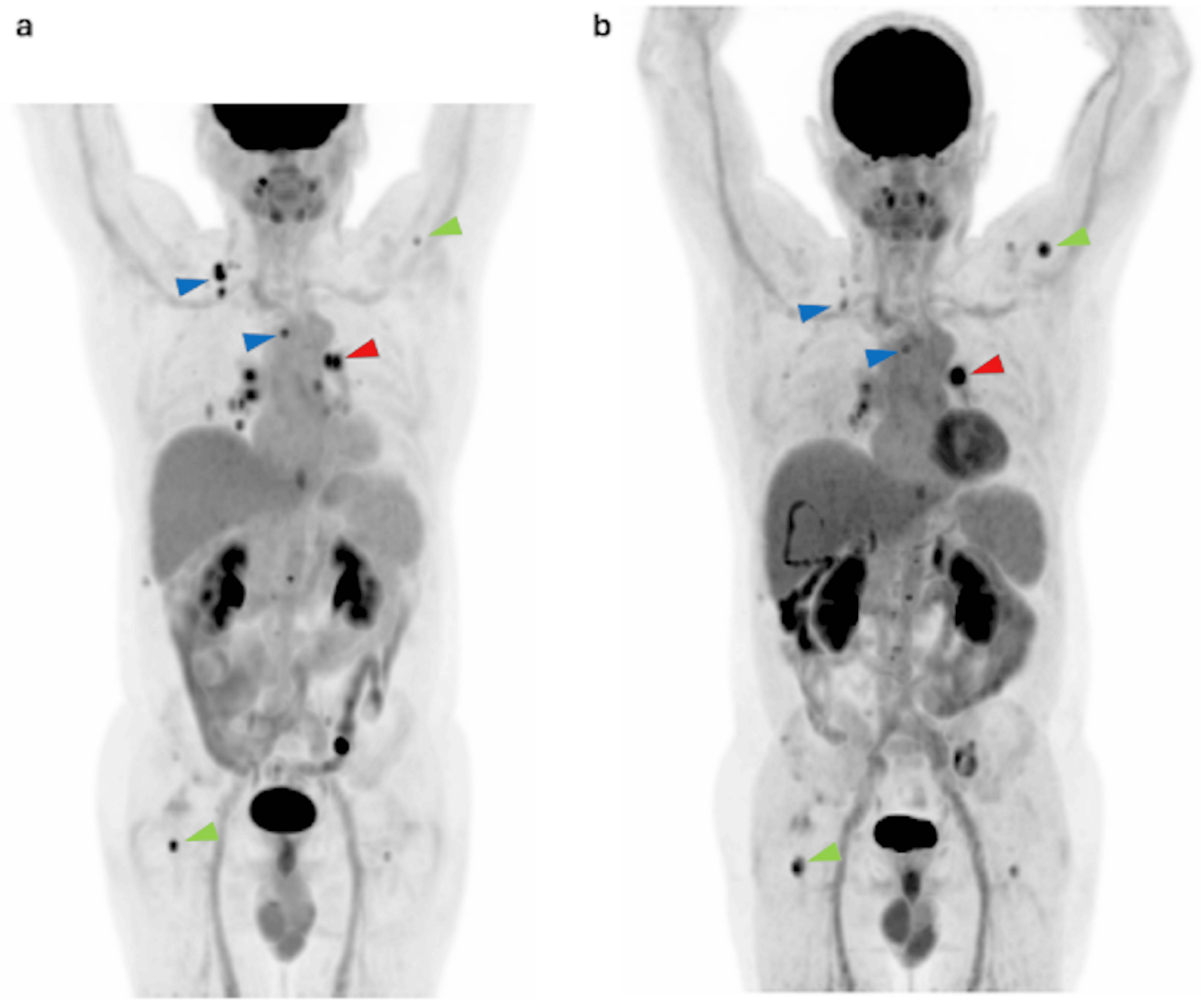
MIP images from FDG-PET/CT studies performed in June 2023 (a) and July 2024 (b). Interval metabolic progression is observed, characterized by increased FDG uptake in a left hilar pulmonary lymph node (red arrowhead) and multiple osseous lesions (green arrowhead), while several cervical and mediastinal lymph nodes show decreased tracer avidity, indicating a mixed metabolic response (blue arrowhead). MIP: Maximum intensity projection; FDG: Fluorodeoxyglucose.
